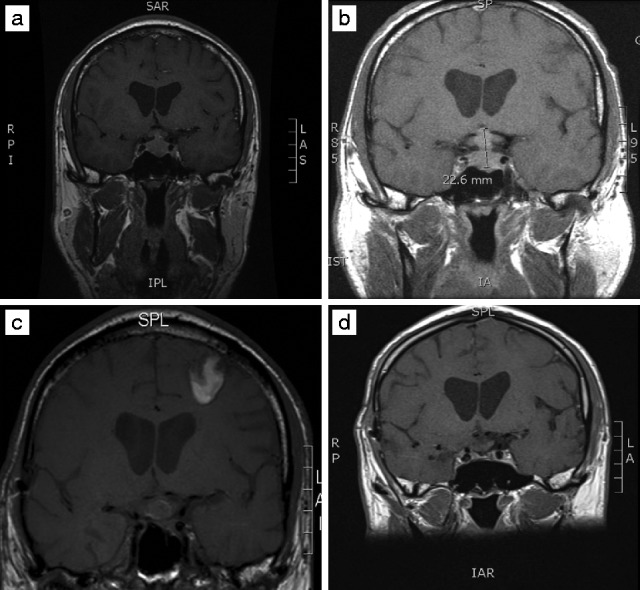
MRI showing T1 post-contrast at the time of diagnosis (a), 7 months after diagnosis (b), 3 months after transcranial surgery (c), and 4 months after conventional radiotherapy (d). Almost 4 years elapsed between the first (a) and the last (d) MRI.
